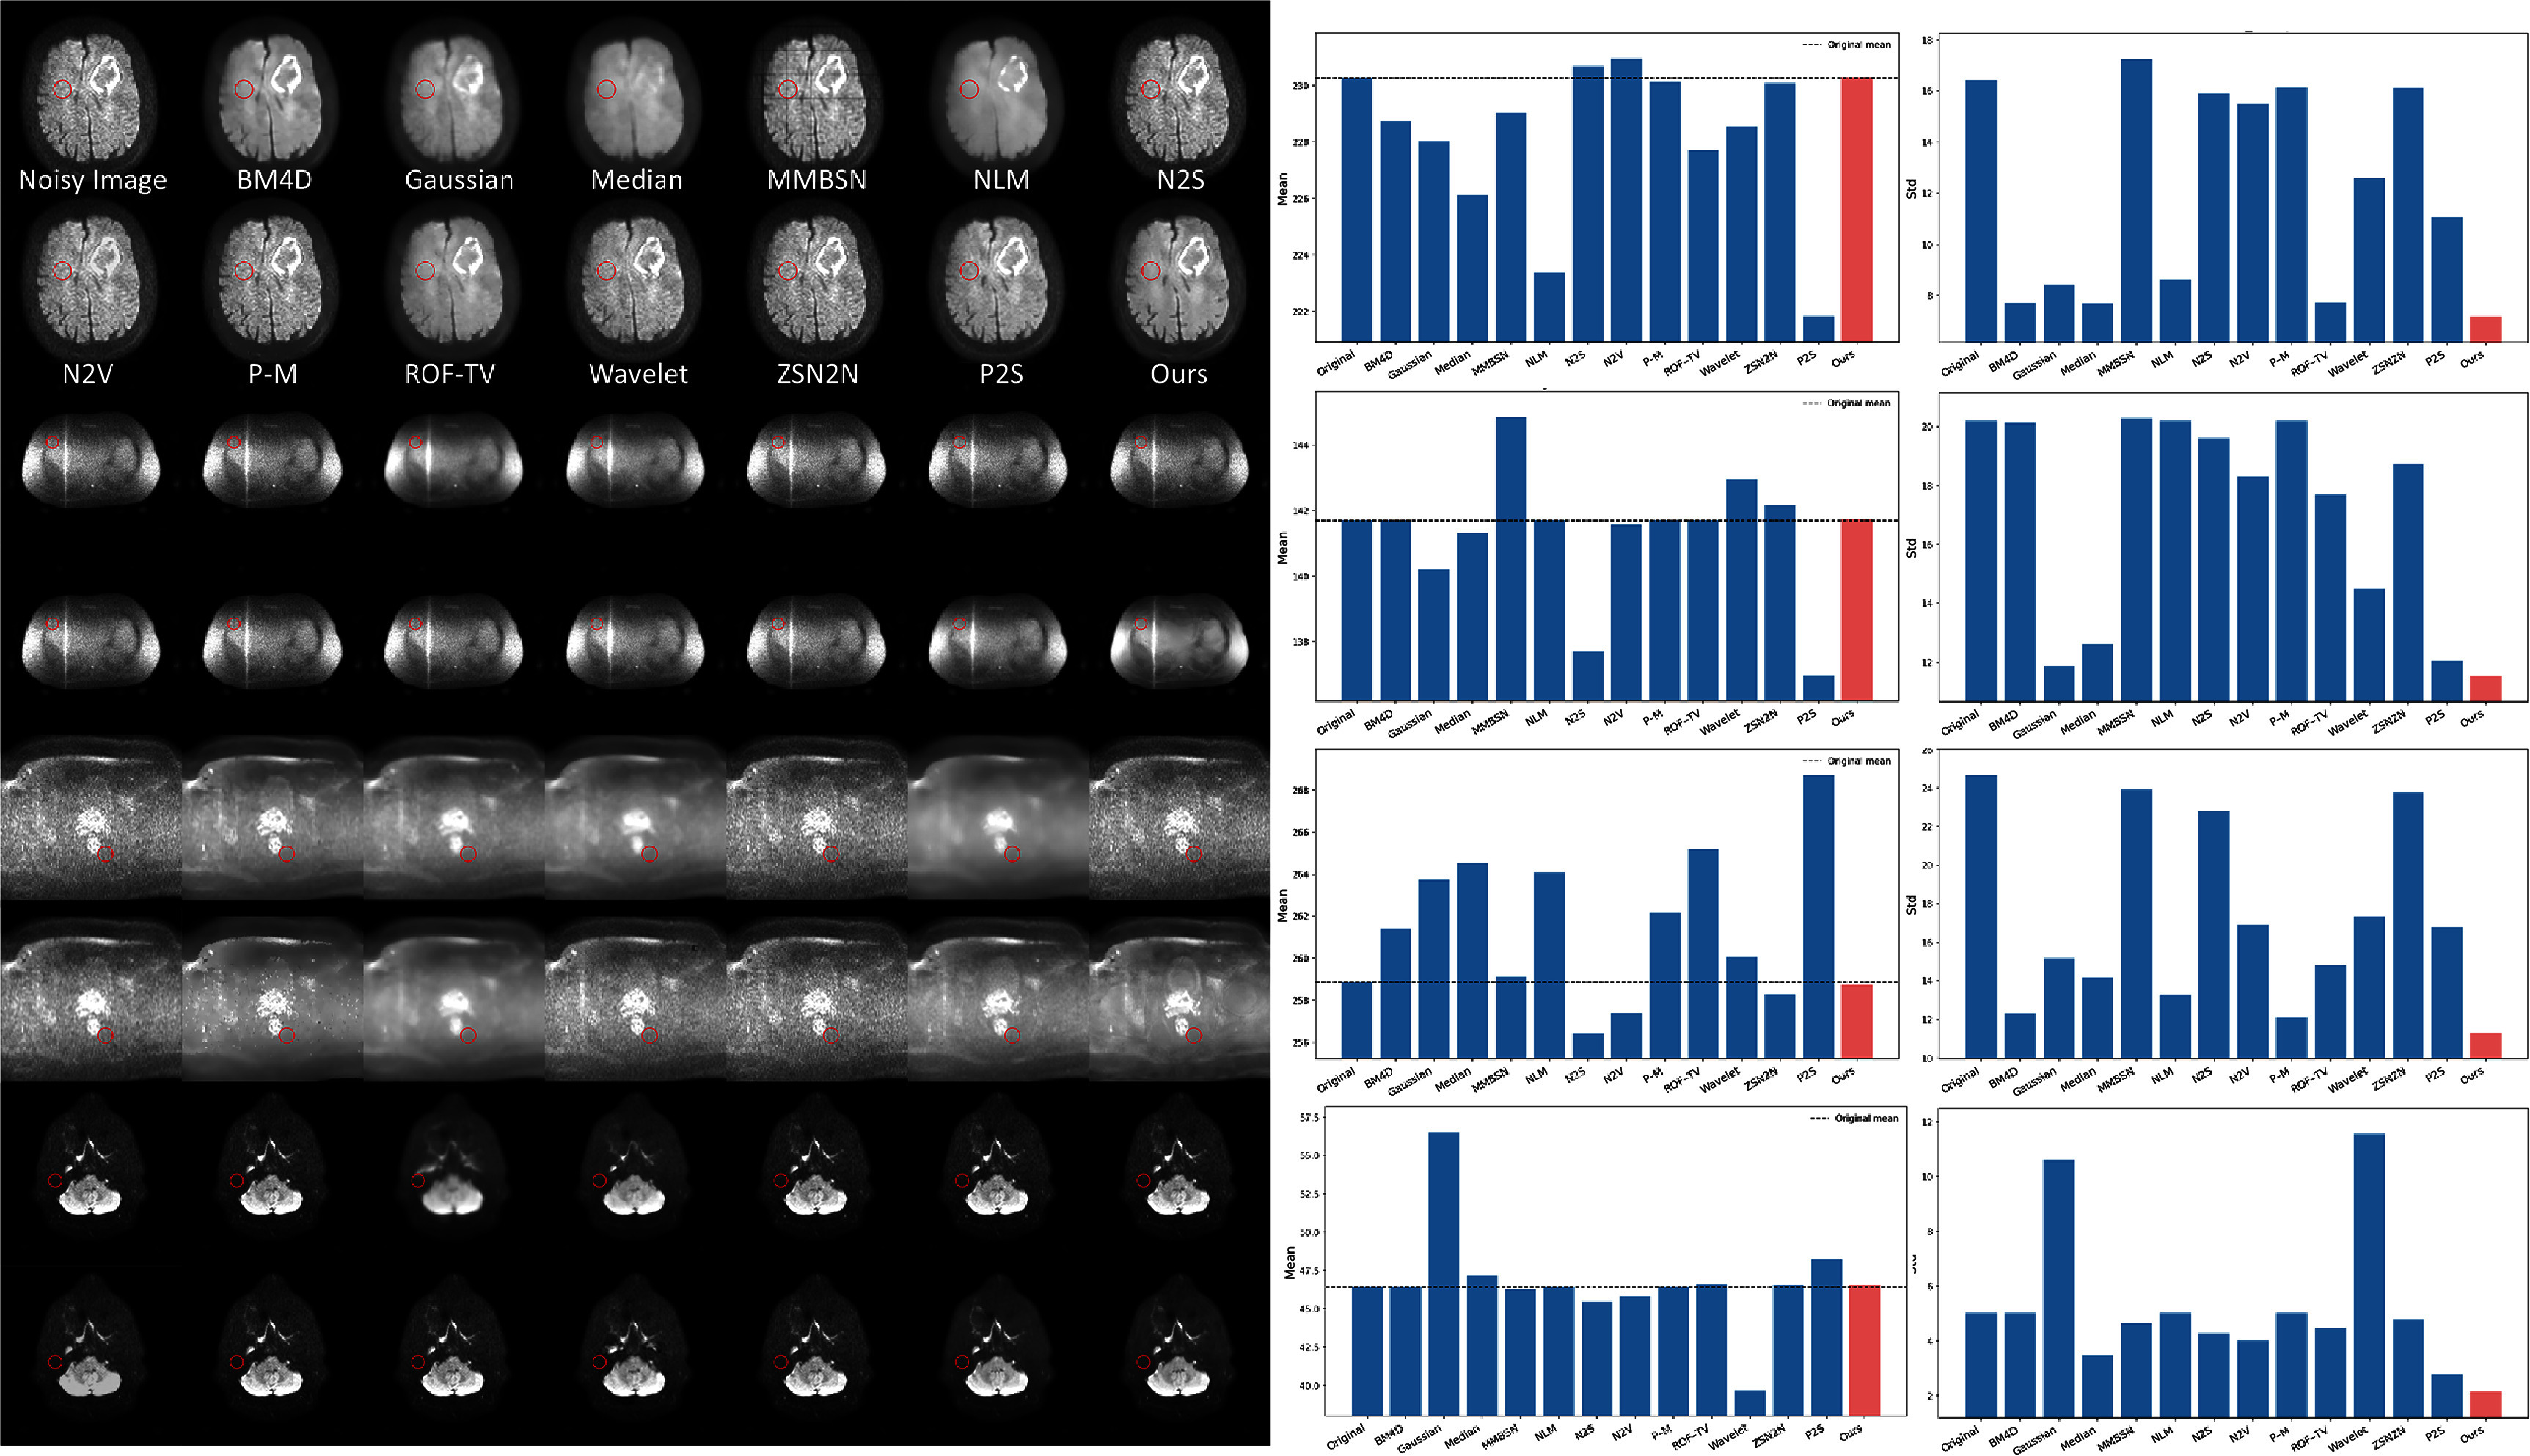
Quantitative statistical analysis of DWI denoising results across different anatomical regions. The figure shows the mean and standard deviation changes of signal intensity in selected regions of interest before and after denoising by different methods for brain, head-and-neck, abdomen, and pelvis. Each subplot demonstrates the noise suppression capability (standard deviation reduction) and signal fidelity (mean preservation) of each method.
